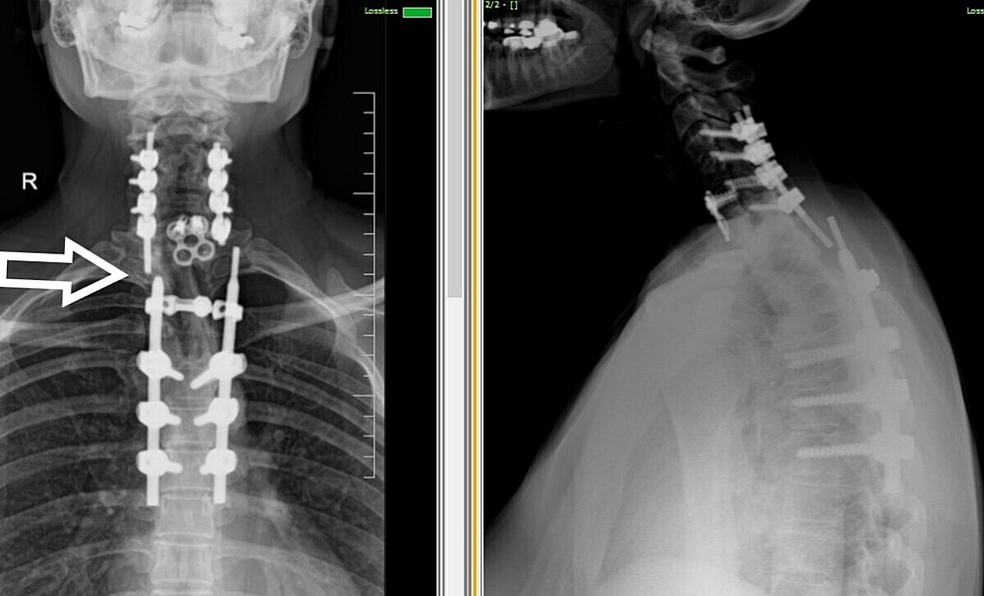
X-ray after seven years with no change in implant status
